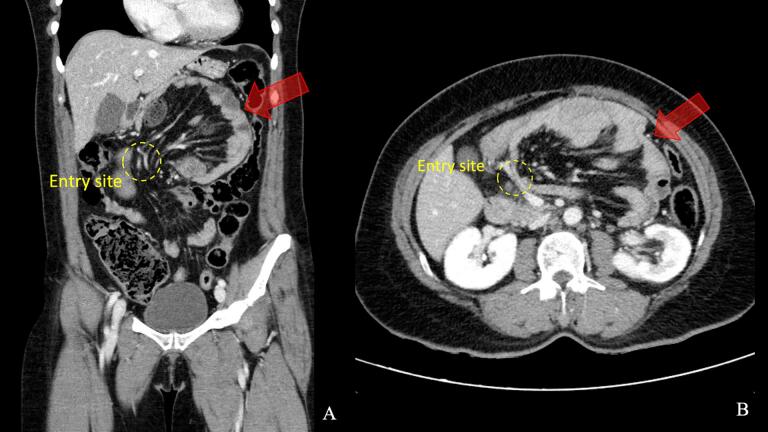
CT scan characteristics of left paraduodenal hernia. A: Coronal view, B: Axial view. Contrast-enhanced CT scan showing a cluster of small bowel loops (arrows) situated between the stomach and pancreas, with associated mass effect.
